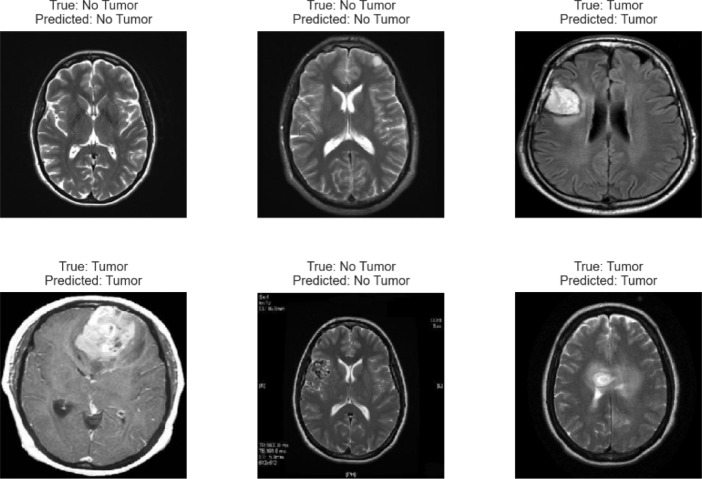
Representative examples for classification process on MRI scans from internal dataset A with the proposed model’s predictions.
